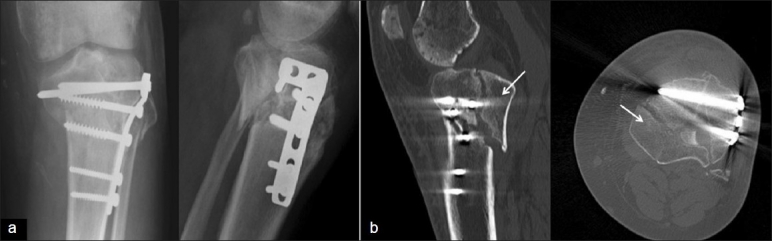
(a) Catastrophic failure of single-plate fixation as the screws have completely missed the coronally based medial condylar fragment. (b) CT scans showing the medial tibial condylar fragment (white arrows) is not stabilized
